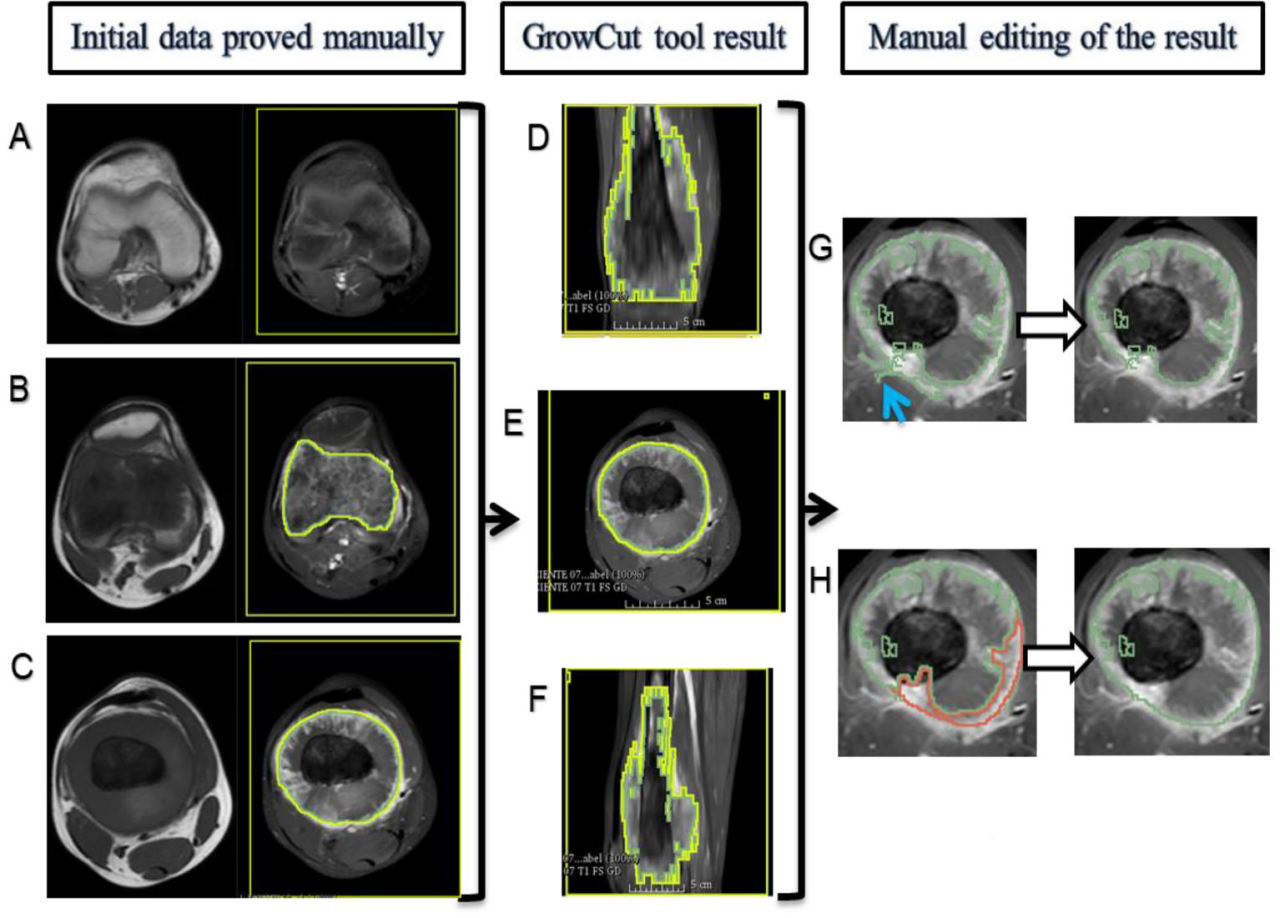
3D-Slicer's GrowCut semiautomatic segmentation steps of a femoral osteosarcoma magnetic resonance imaging case. In the first column (A, B, and C), the T1WI sequence images are on the left and the T1WI FS GD sequence images are on the right. In the second (D, E, and F) and third columns (G and H), there are only T1WI FS GD sequence images. A, Yellow rectangular area of background tissue beyond the inferior margin of tumor. B and C, Area of interest from the tumor tissue within green mark and background tissue within yellow mark in the extremities of the tumor (B) and in the middle portion of the tumor on the longitudinal axis (C). D, E, and F, Segmented volume of interest within green mark after GrowCut tool processing in coronal (D), axial (E), and sagittal (F) planes. G and H, Manual editing of GrowCut tool excluding areas from the volume of interest (within green mark) that do not contain tumor tissue, as indicated with the blue arrow in (G), and including areas with tumor tissue in the volume of interest, as shown in area marked in red (H).
